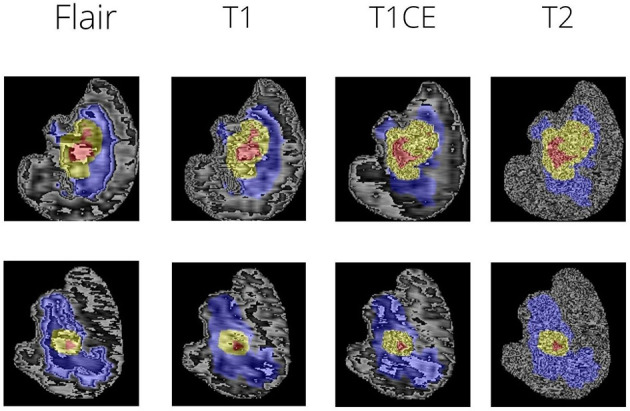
The different views of brain MRI slices with annotations.
